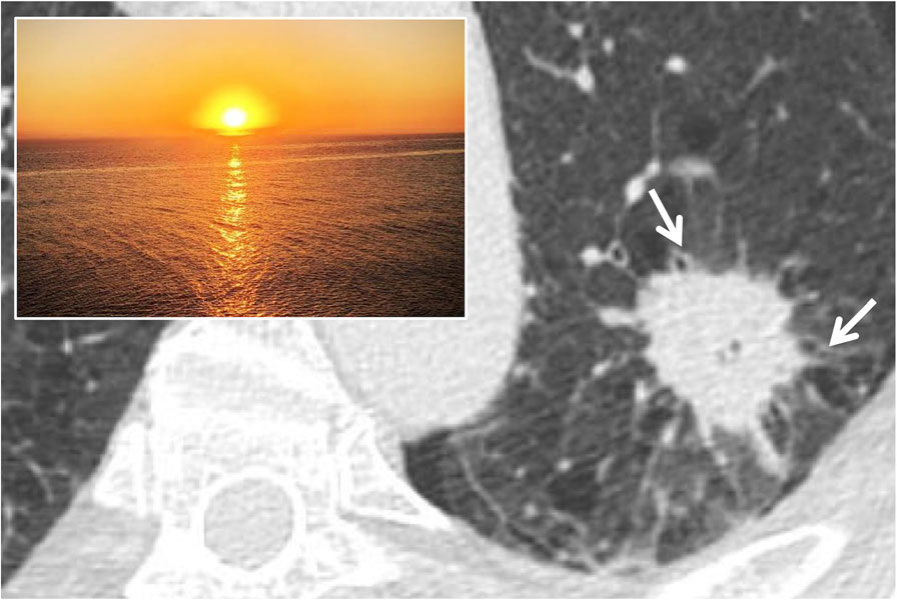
Sunburst sign. A CT image of a 61-year-old female affected by lung adenocarcinoma. The parenchymal neoplastic lesion shows spiculated and irregular margins, resembling the appearance of a “sunburst”: the rays or the speculated margins are constituted by the distorted blood vessels and/or by thickened septa that surround the pulmonary mass
